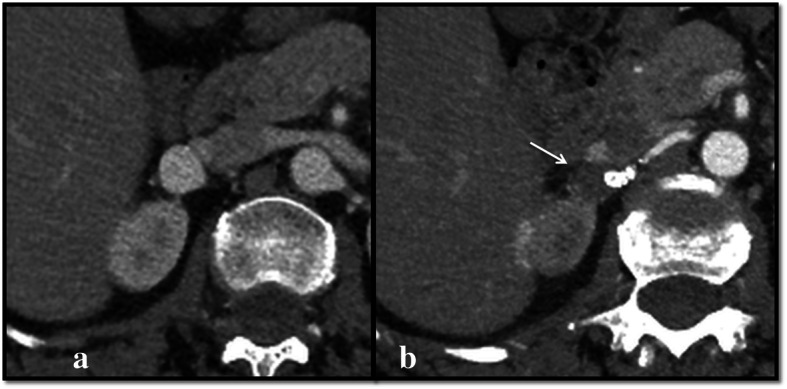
a, b Axial pre-operative (a) CTA scans show the presence of a VAA of the right renal artery (Patient 1), which appears completely excluded (arrow) at the 12-month follow up (b)
